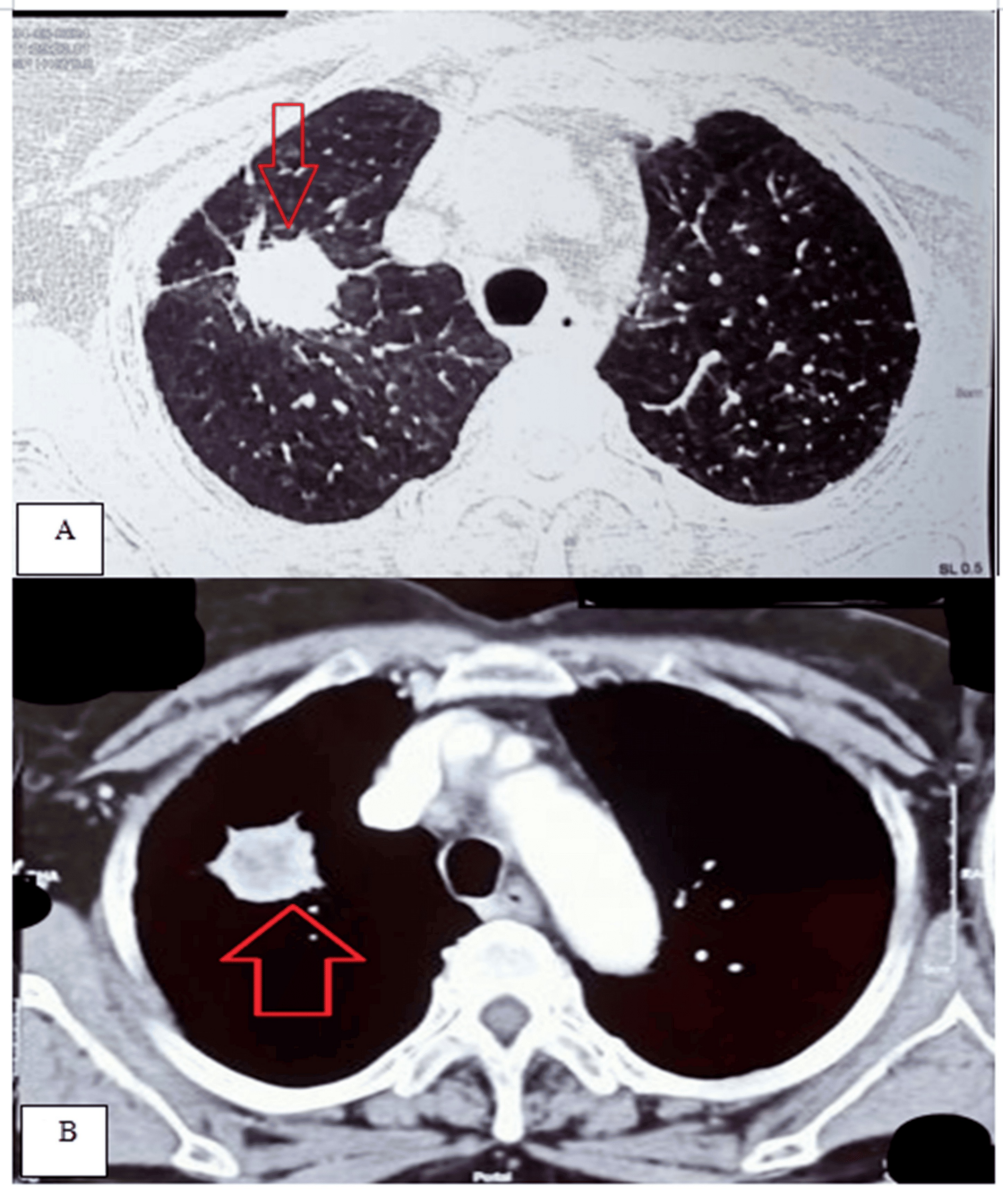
A) CT thorax (axial slice) showing soft tissue density lesion in the right upper lobe of the lung with heterogeneous enhancement (marked with a red arrow). B) CECT thorax (axial slice) showing irregular soft tissue density lesion in the right upper lobe of the lung with spiculated margins (marked with a red arrow)
